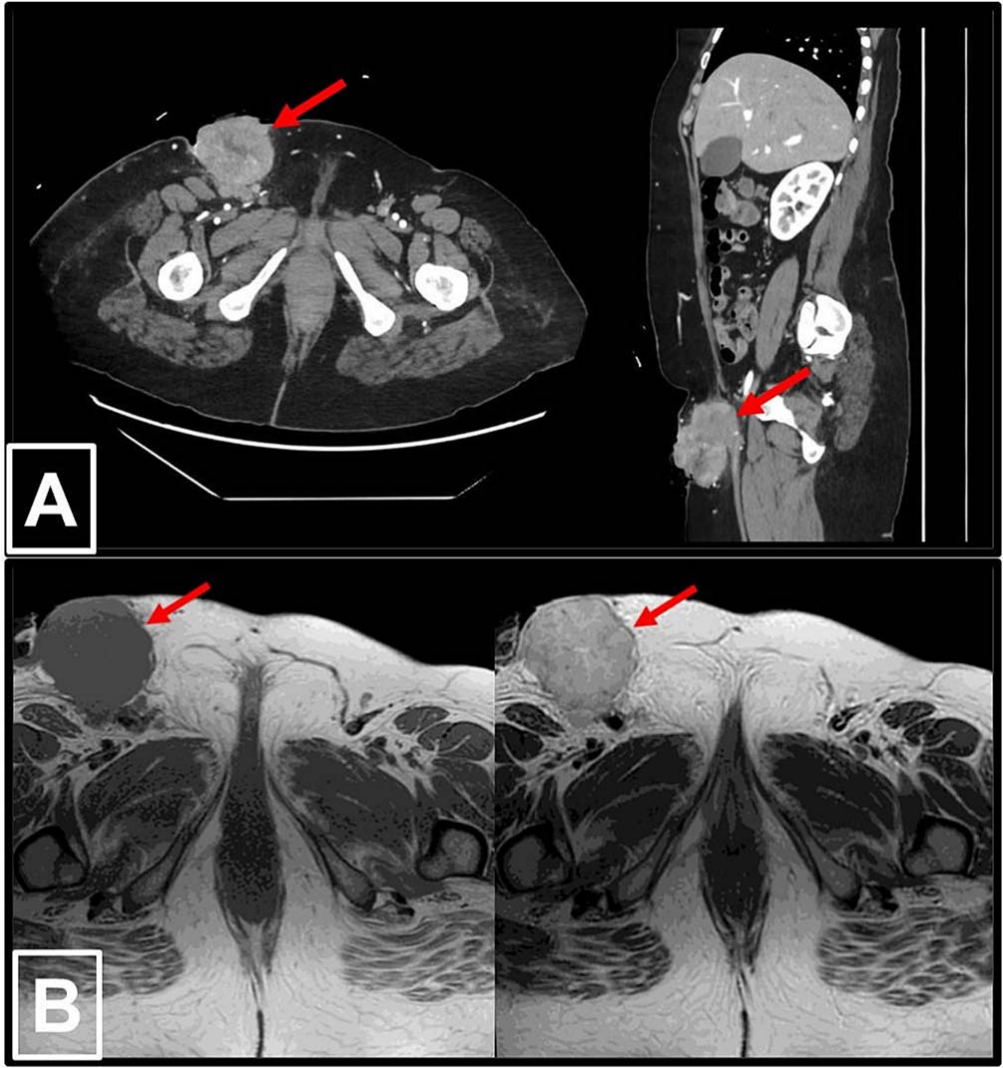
Radiological imaging of the right inguinal mass. (a) Contrast-enhanced CT scans of the abdomen and pelvis in axial (left) and sagittal (right) views, demonstrating a pathologically enlarged necrotic right inguinal lymph node (red arrow), measuring 5.3 × 6.2 × 8.5 cm. The mass is ulcerating into the inguinal skin and is in very close proximity to the adjacent inguinal vessels. (b) MRI of the pelvis in T1-weighted (left) and T2-weighted (right) sequences, again revealing the pathologically enlarged necrotic right inguinal lymph node (red arrow).
